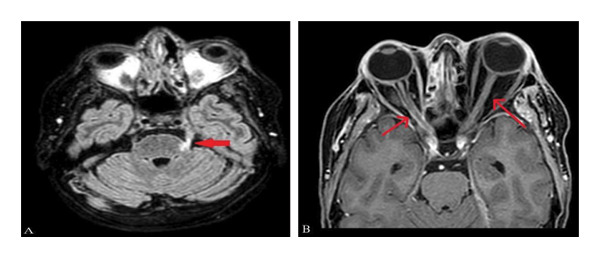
Brain MRI showing the findings suggestive of infectious neuritis involving the intracranial portion of the left trigeminal nerve (A) and bilateral optic nerves (B).
